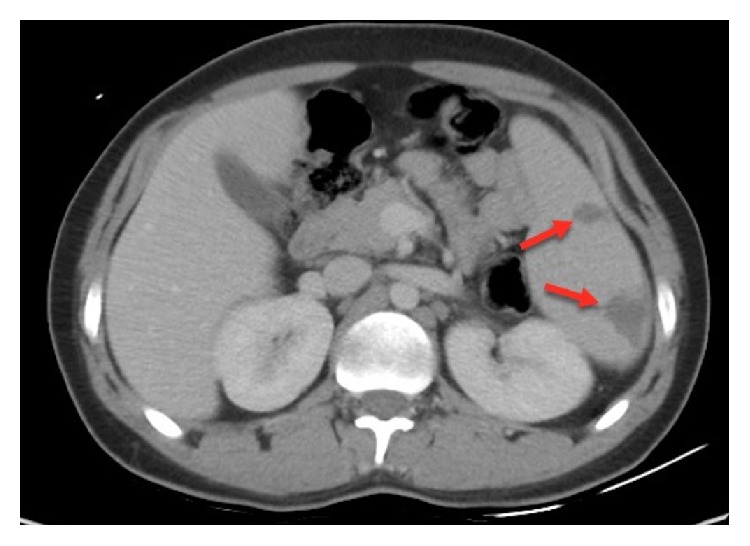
Abdomen CT without contrast showing multiple hypodense splenic lesions.
